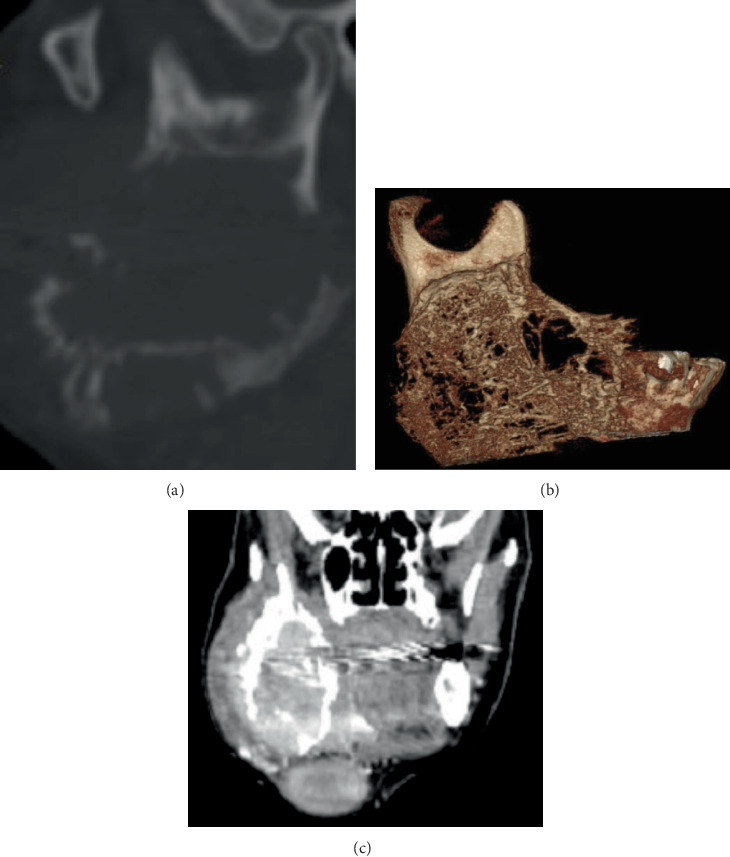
Superior extension of the lesion presented on (a) the sagittal computed tomography cut and (b) three-dimensional segmented right mandible view generated by OnDemand3D App software (Cybermed Inc., Seoul, Korea). (c) Inferior extension of the lesion presented on a raw coronal computed tomography plan.
